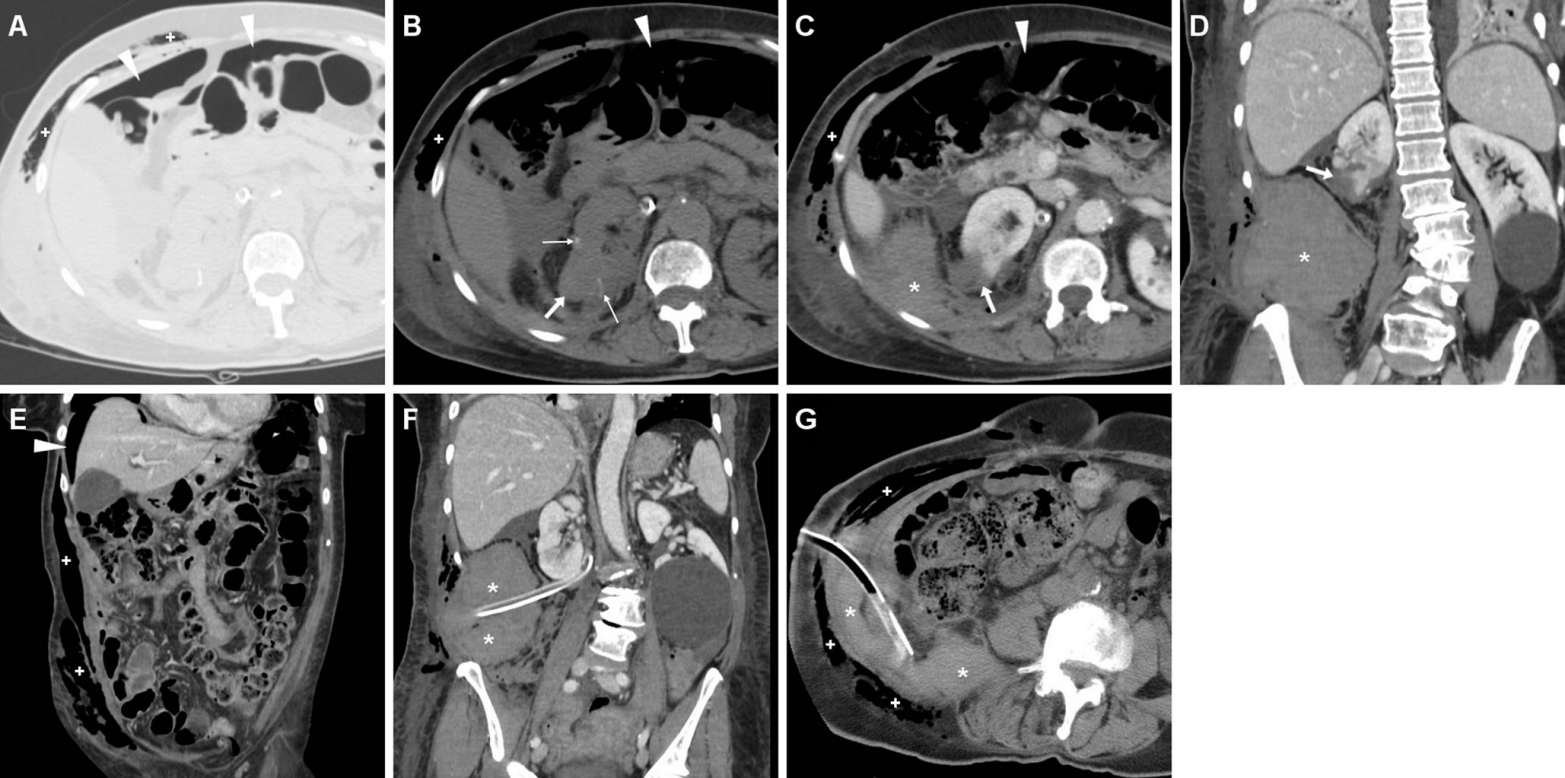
Two days after L-NSS for T1N0M0 RCC of the right kidney, the same patient as in Fig. 1a, b suffered from abdominal pain and progressive blood loss (nadir haemoglobin 11.5 g/dL), with stable vital signs. Urgent CT images viewed at lung window settings (a) showed moderate pneumoperitoneum (*arrowheads*) and subcutaneous emphysema of the abdominal wall (+). Unenhanced (b) and post-contrast (c–f) image showed the renal resection site (*arrows* in b–d) with sutures (*thin arrows*), a sizeable haematoma centred in the posterior para-renal space (*) causing kidney displacement, without active bleeding. Coronal CT reformation (f) confirmed massive subcutaneous emphysema (+) and pneumoperitoneum (*arrowhead*). Following conservative treatment and drainage tube repositioning, repeated CT 5 days later (g) showed persistent subcutaneous emphysema (+), decreased retroperitoneal blood collection (*)
